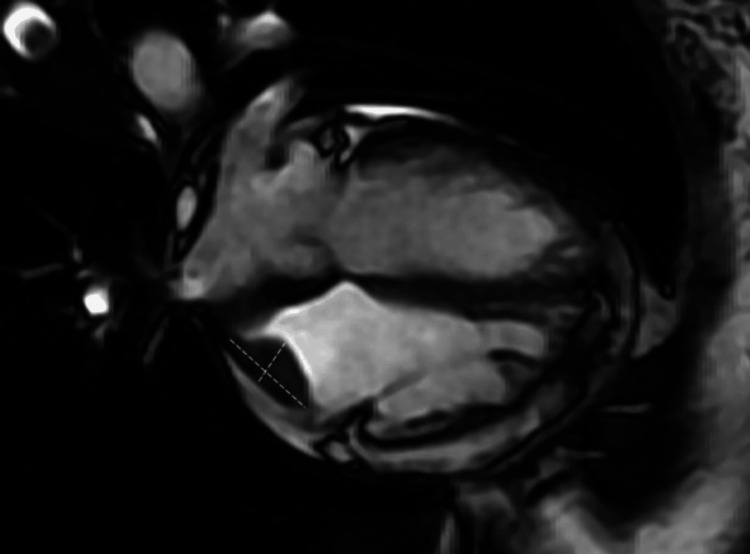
Preoperative cardiac magnetic resonance imaging (MRI). Horizontal long-axis (four-chamber) view; dashed calipers denote the measured dimension (3.0 cm × 1.5 cm) of the mass in the right atrium adherent to the inferoposterior wall.
